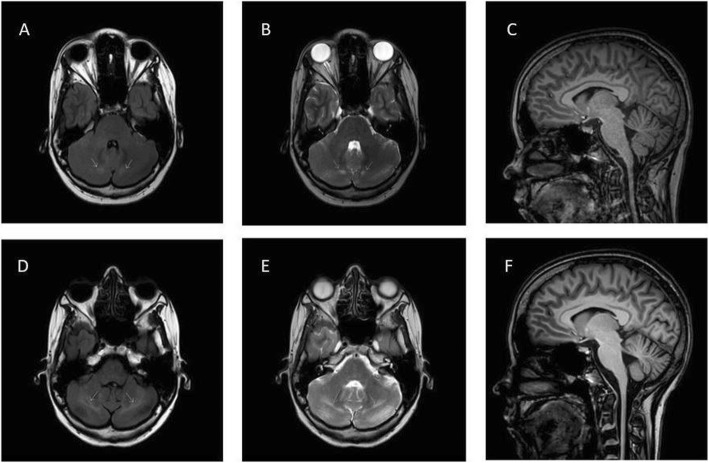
Brain MRI of the patient. a (FLAIR horizontal), b (T2 horizontal) and c (sagittal horizontal) are MRI examinations performed in 2008, while d (FLAIR horizontal), e (T2 horizontal) and f (sagittal) was performed in 2016. The hyperintense signs (white arrows) in the cerebellum are clearly visible on the images and show progression between the two examinations. Cerebellar, medulla oblongata, and cervical spinal cord atrophy can also be observed
